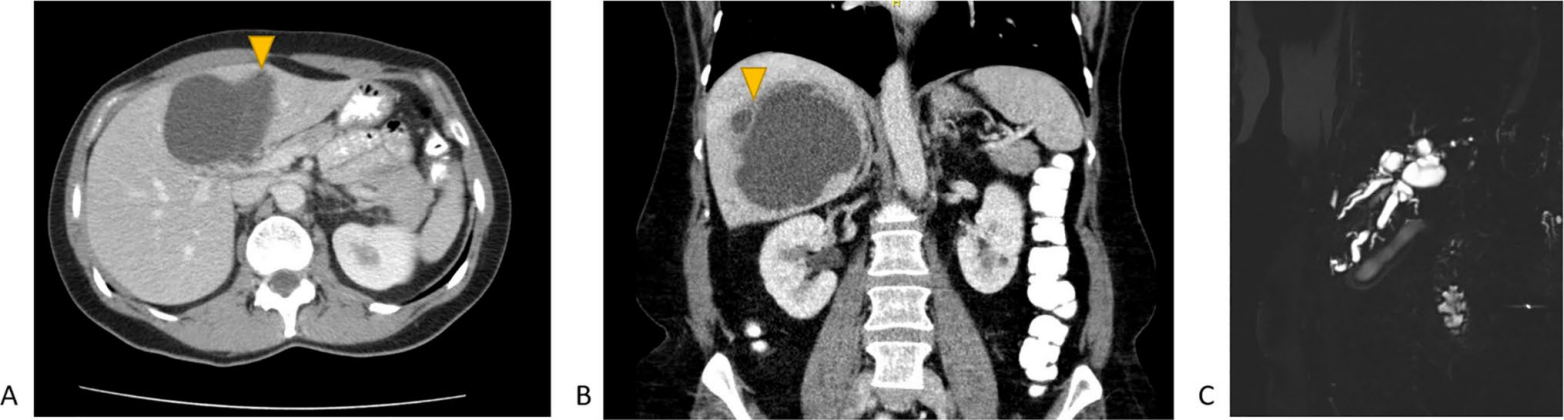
Representative CT and MRI images of patients in whom imaging assessment according to the EASL-guidelines did not correspond to the final pathological diagnosis A. CT-scan of a 52-year-old female patient with a liver cyst with low suspicion of MCN-L based on imaging assessment according to the EASL-guidelines. Final pathological diagnosis was MCN-L. B and C. CT-scan and MRI images of a 59-year-old patient with a liver cyst with high suspicion of MCN-L based on imaging assessment according to the EASL-guidelines. Final pathological diagnosis was simple hepatic cyst. A. The cyst measuring 8.7 cm, located in segment 4b, showed no nodularity or thick septations (no major worrisome features). Thin septations and less than three co-existent cysts were seen (two minor worrisome features). No upstream biliary dilatation, haemorrhage, or perilesional perfusional change were seen. B and C. The cyst measuring 10.6 cm in diameter, located in the right hemiliver, showed thick septations (one major worrisome feature), indicated by the yellow arrow. The cysts showed upstream biliary dilation (figure C) and less than three co-existent hepatic cysts (two minor worrisome features). No perilesional perfusional change or haemorrhage was observed
